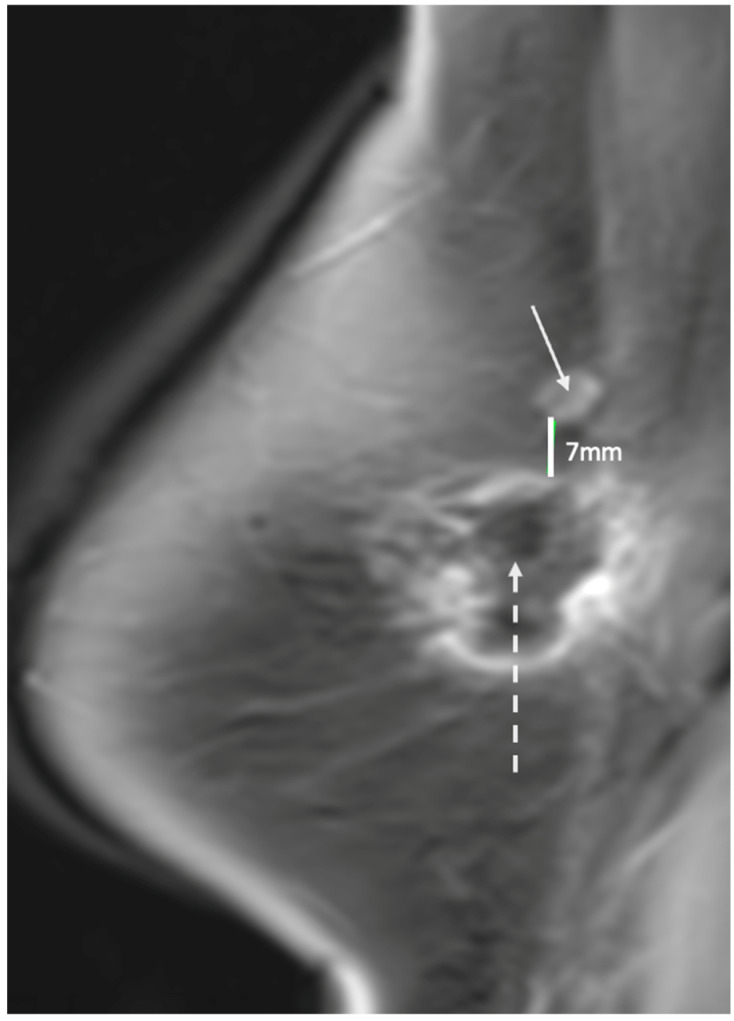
Contrast enhanced breast MRI showing new area of suspicious enhancement (indicated by solid arrow) suggestive of a recurrence 7 mm superior to spherical cryoablation zone of necrosis (indicated by broken arrow).
